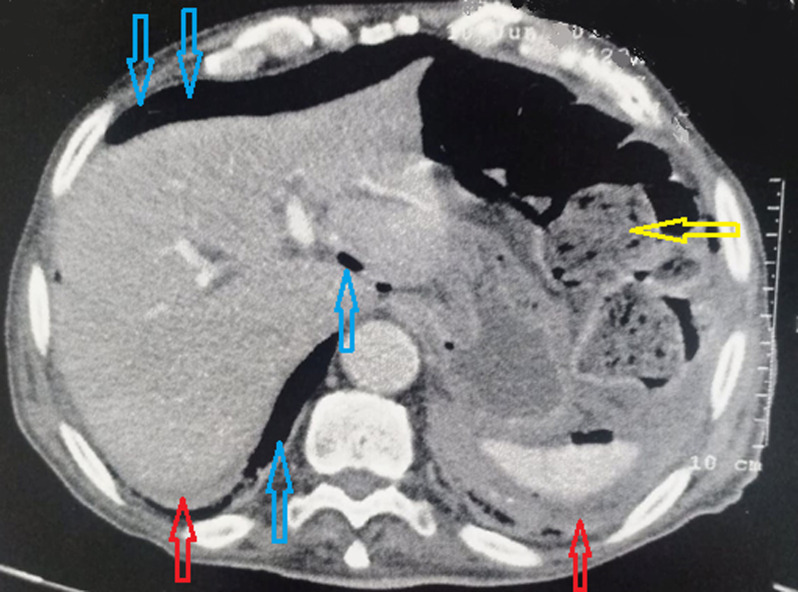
abdominal CT revealing pneumo peritoneum (blue arrow), and moderate free fluid (red arrow), and fecal impaction of the large bowel (yellow arrow)
